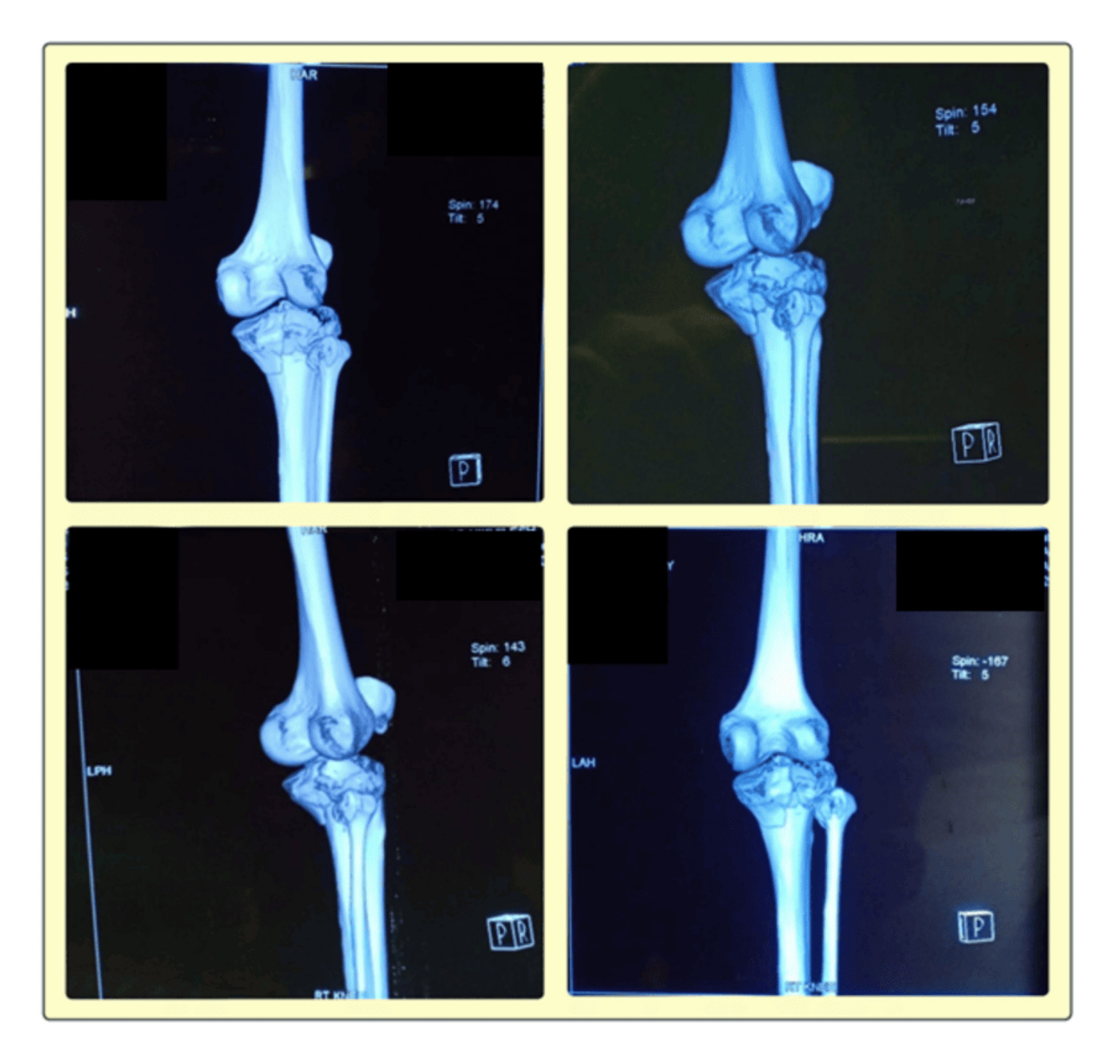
3D CT scan images reveal a comminuted intra-articular fracture of the proximal tibia with significant displacement. These scans provide critical insights into the fracture pattern, joint involvement, and surgical planning, for optimal fixation and functional recovery.
